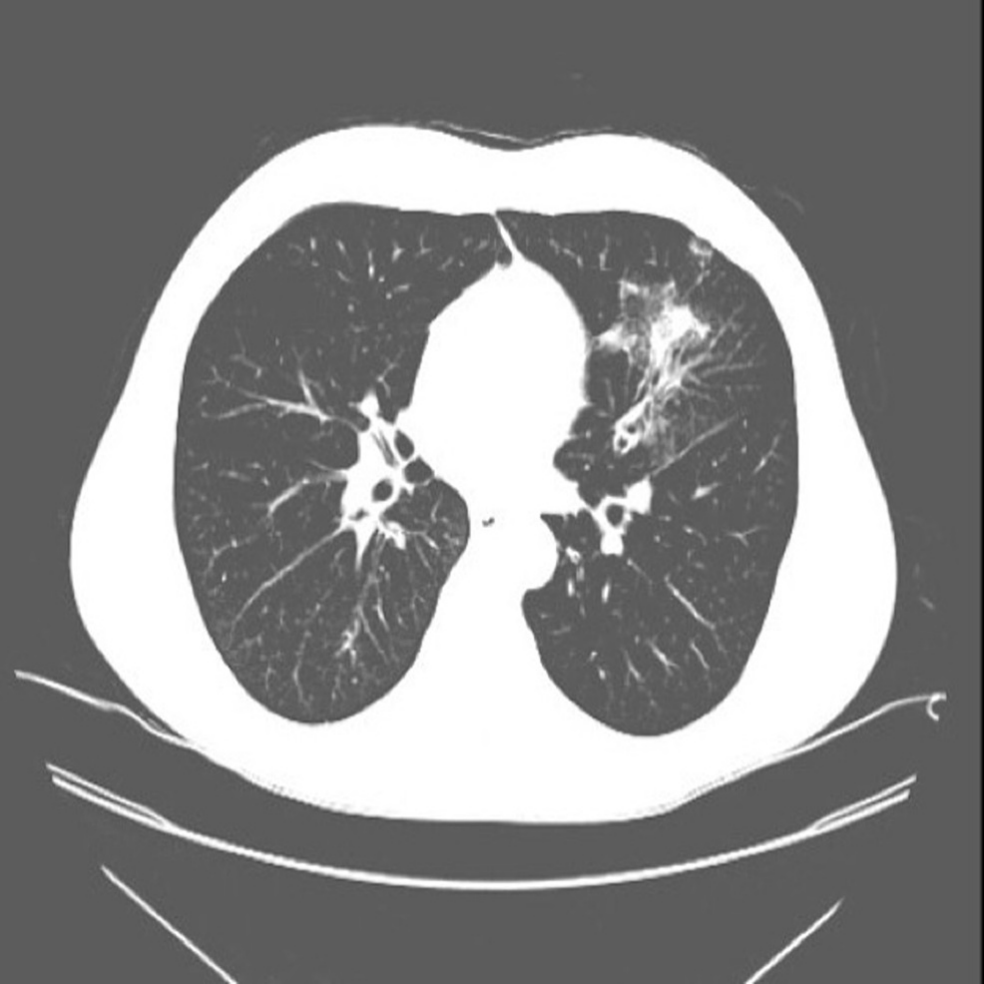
Reticulonodular infiltrates on the left lower lobe on CT
